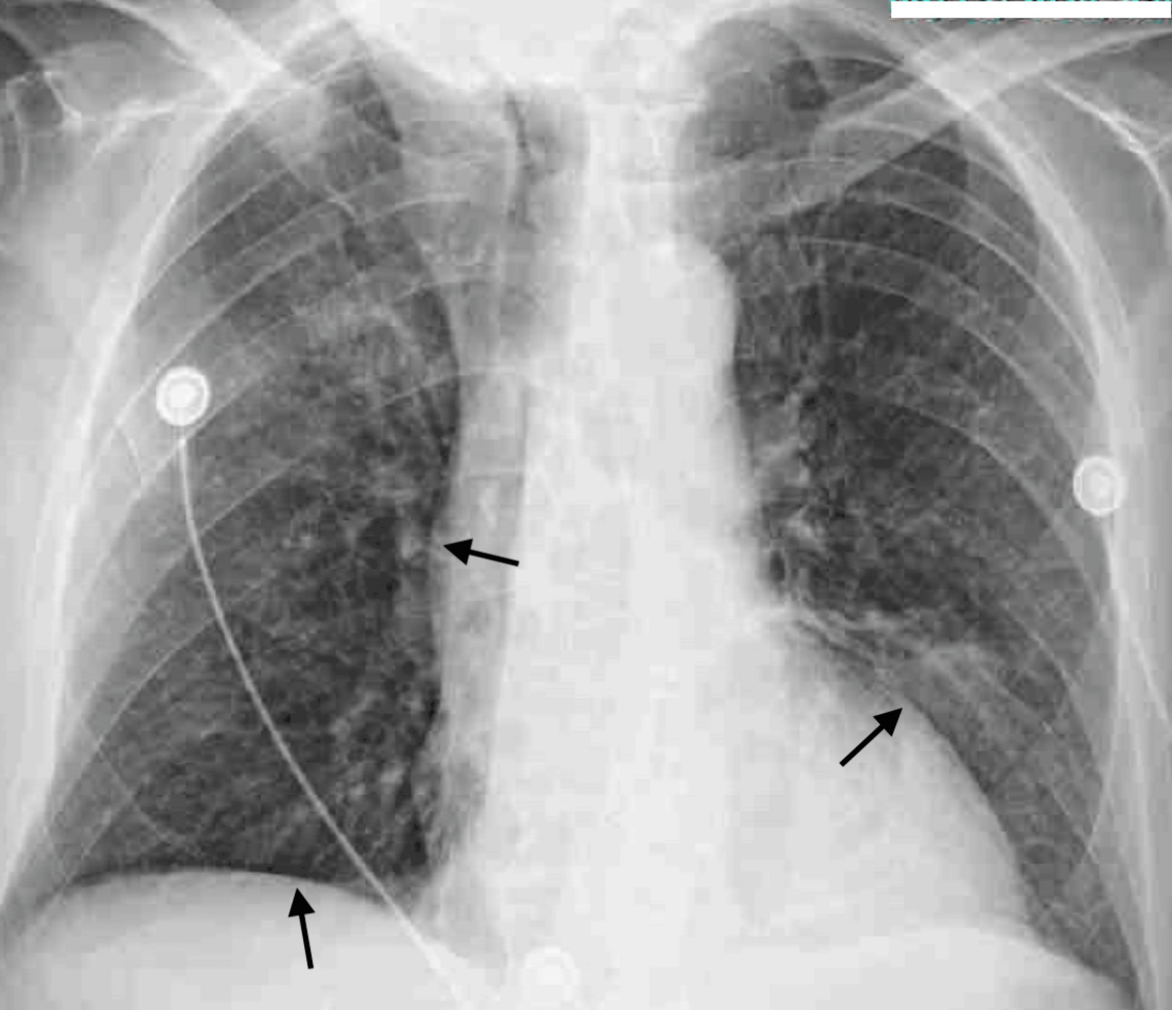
Post-treatment CXR AP view CXR AP done after finishing pulse dose steroids for three days. Arrows indicate improvement in haziness seen previously. CXR: chest X-ray, AP: anteroposterior
